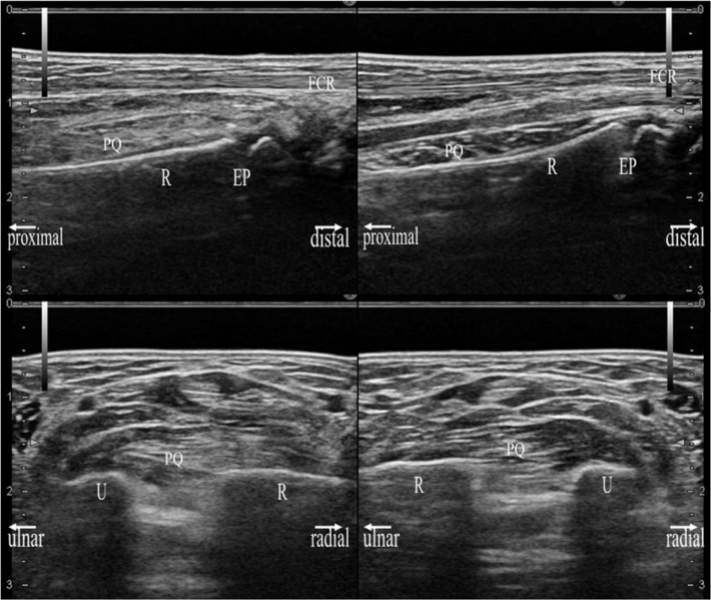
Sonographic images of an 11-year-old girl with a diagnosis of distal radius contusion of the left hand. Sonographic images in the longitudinal (upper image) and transverse (lower image) planes were obtained using a 17-MHz transducer. Each image shows a side-by-side comparison of the right hand (right image) and left hand (left image). The PQ of the left hand is locally thickened with hyperechogenicity on the radial side. The PQ shows a convex appearance in either hand. EP: epiphyseal plate.
